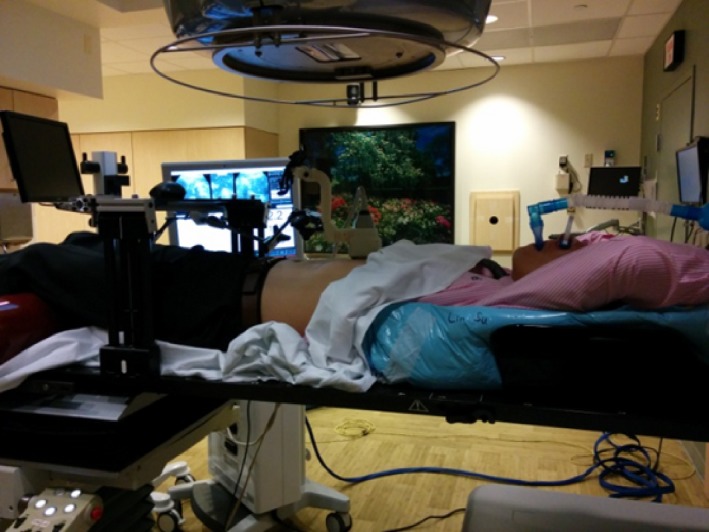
Volunteer setup in the treatment room with ultrasound system and ABC. The gantry rotated around the volunteer to assess clearance.
